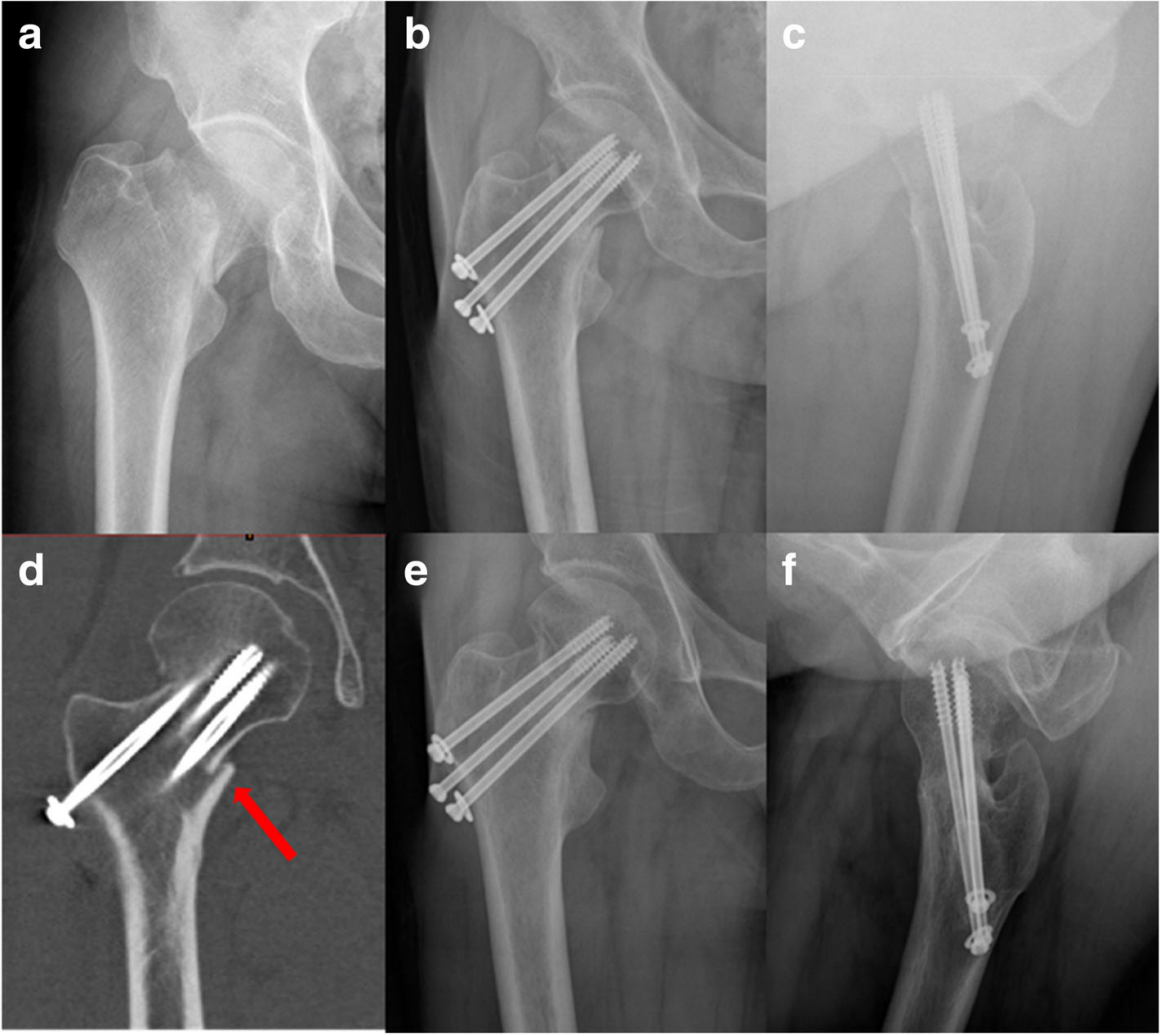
AP view of femoral neck fracture in a 53-year-old male (a). The fracture was reduced with an inferior cortex support pattern (b, c). CT showed cortex buttress (red arrow, d). Although gradually displaced to varus (> 10°) and shortening of the femoral neck, the fracture eventfully united and the patient restored full-weight-bearing walk in 6 months postoperatively (e, f)
